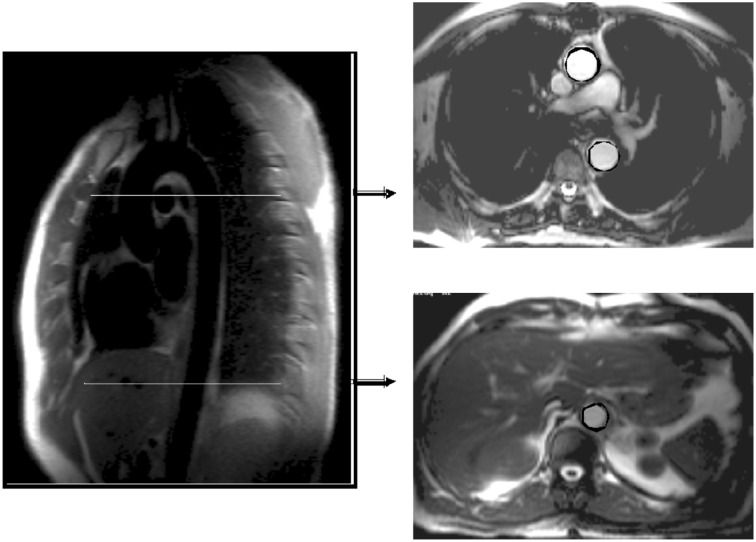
Plane selection for measures of distensibility and pulse wave velocity in ascending, proximal, descending, and distal descending aorta obtained from cardiac magnetic resonance imaging (measured at the Oxford Centre for Clinical Magnetic Resonance Research).
